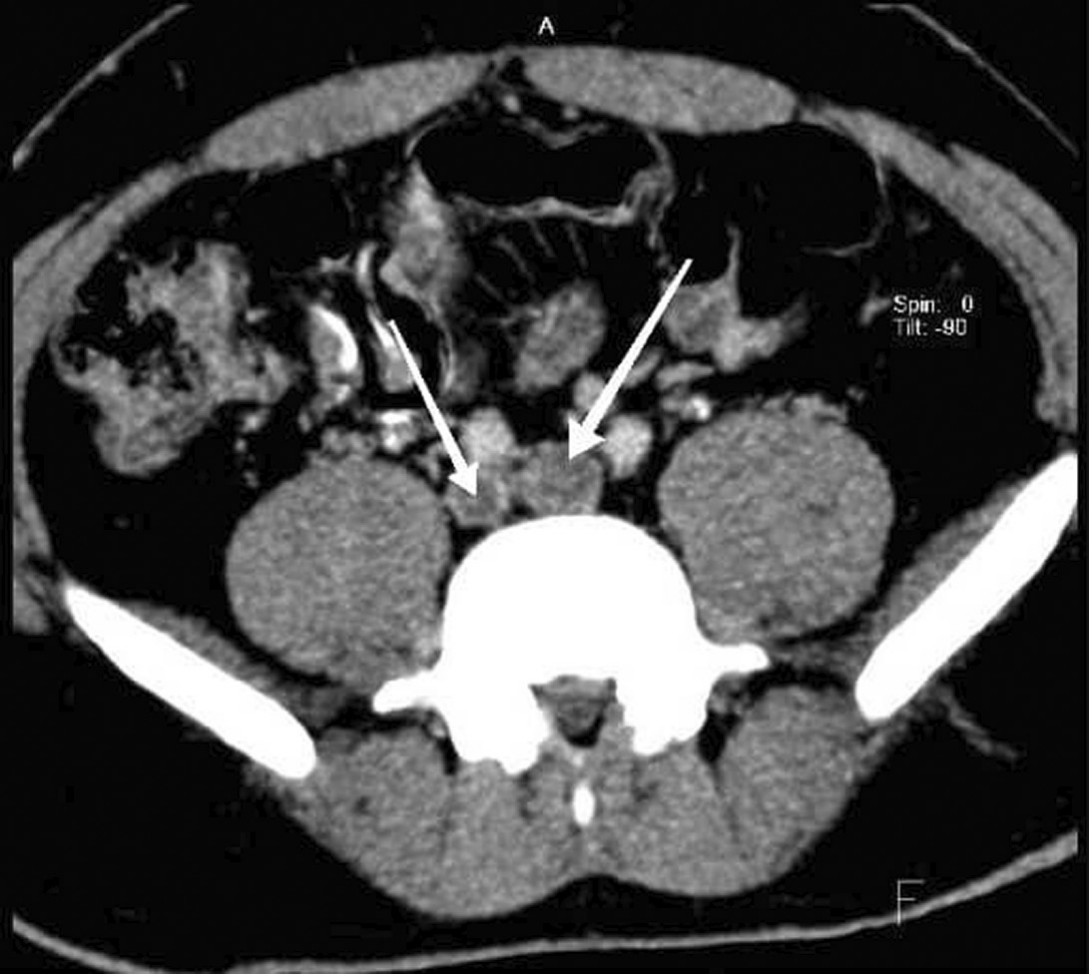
Axial CT delayed phase images, recent thrombosis in both common iliac veins (arrows)
